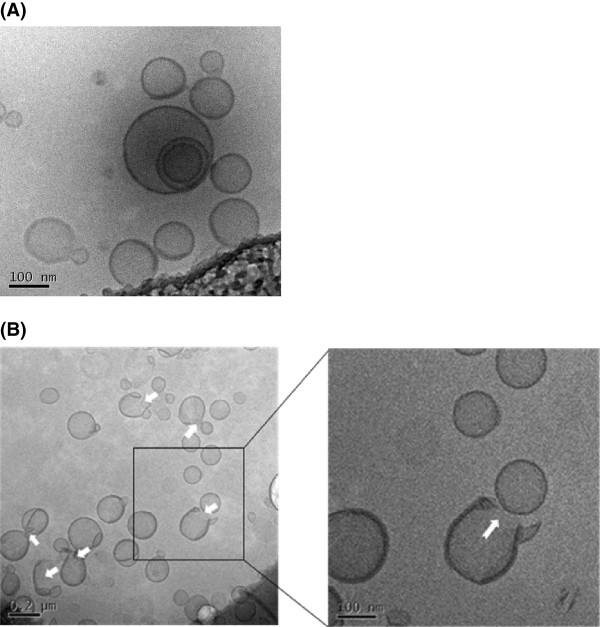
Cryo-TEM images of (A) GdSL3 and (B) ultrasound-irradiated GdSL3. GdSL3 was irradiated by 20-kHz ultrasound for 5 min at an intensity of 63.5 W/cm^2^. The arrows indicate the snapped liposomal membrane.
